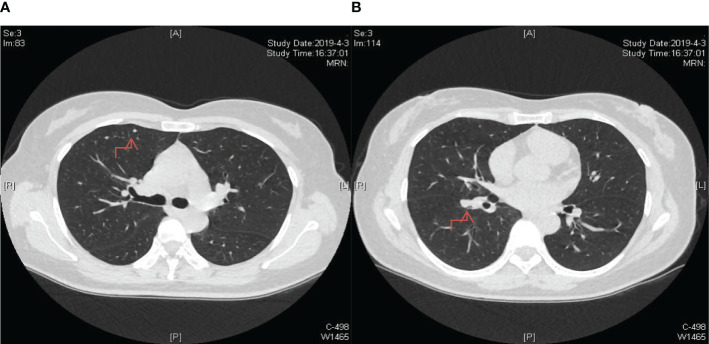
Post-completion of chemotherapy sessions, a follow-up chest CT scan revealed an augmented count and dimensions of nodules within bilateral lungs, indicating the emergence of lung metastases. (A) The lesion in the upper lobe of the right lung; (B) The lesion near the oblique fissure in the lower lobe of the right lung.
